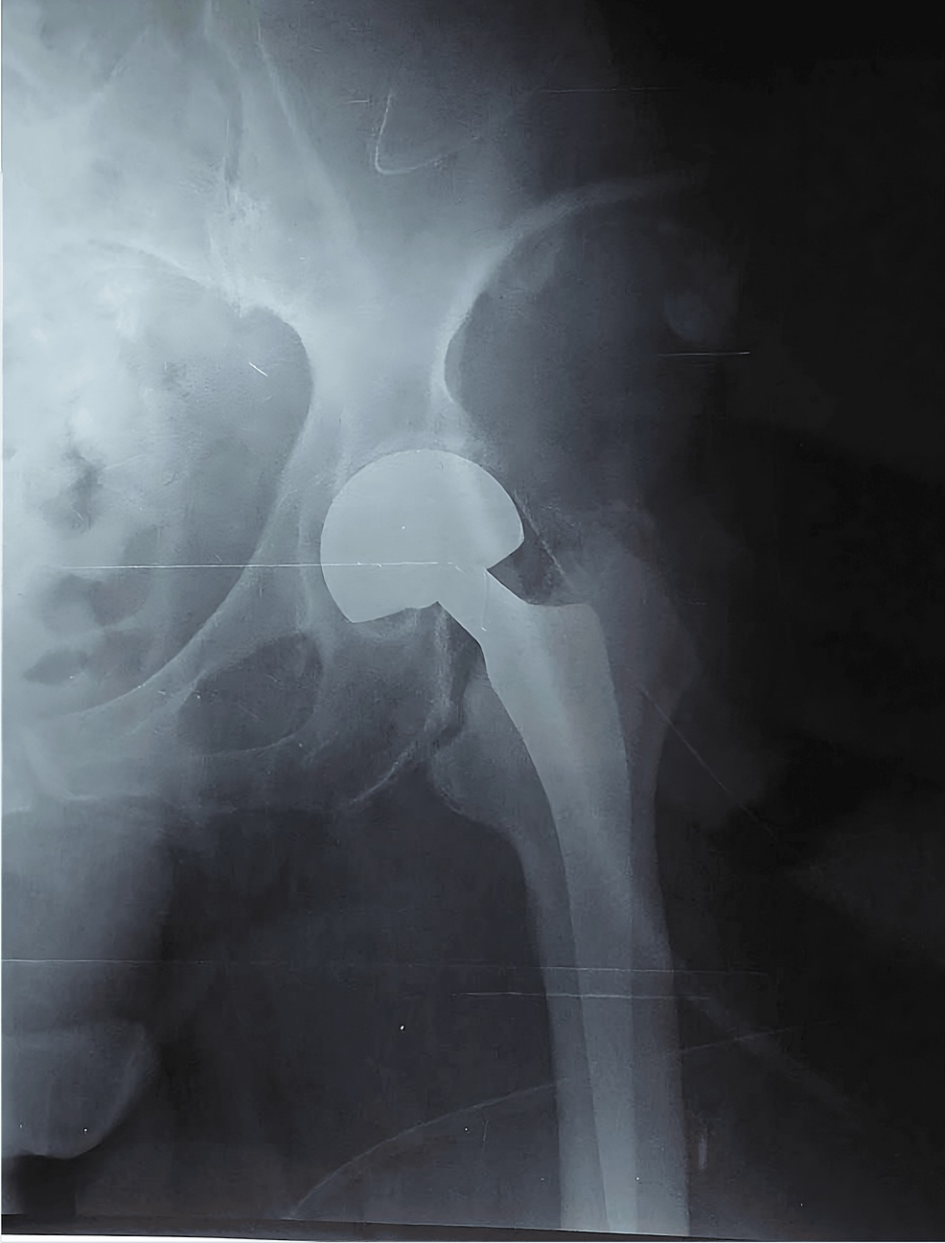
Postoperative left hip radiograph. Good positioning of the left hip hemiarthroplasty components was confirmed.
